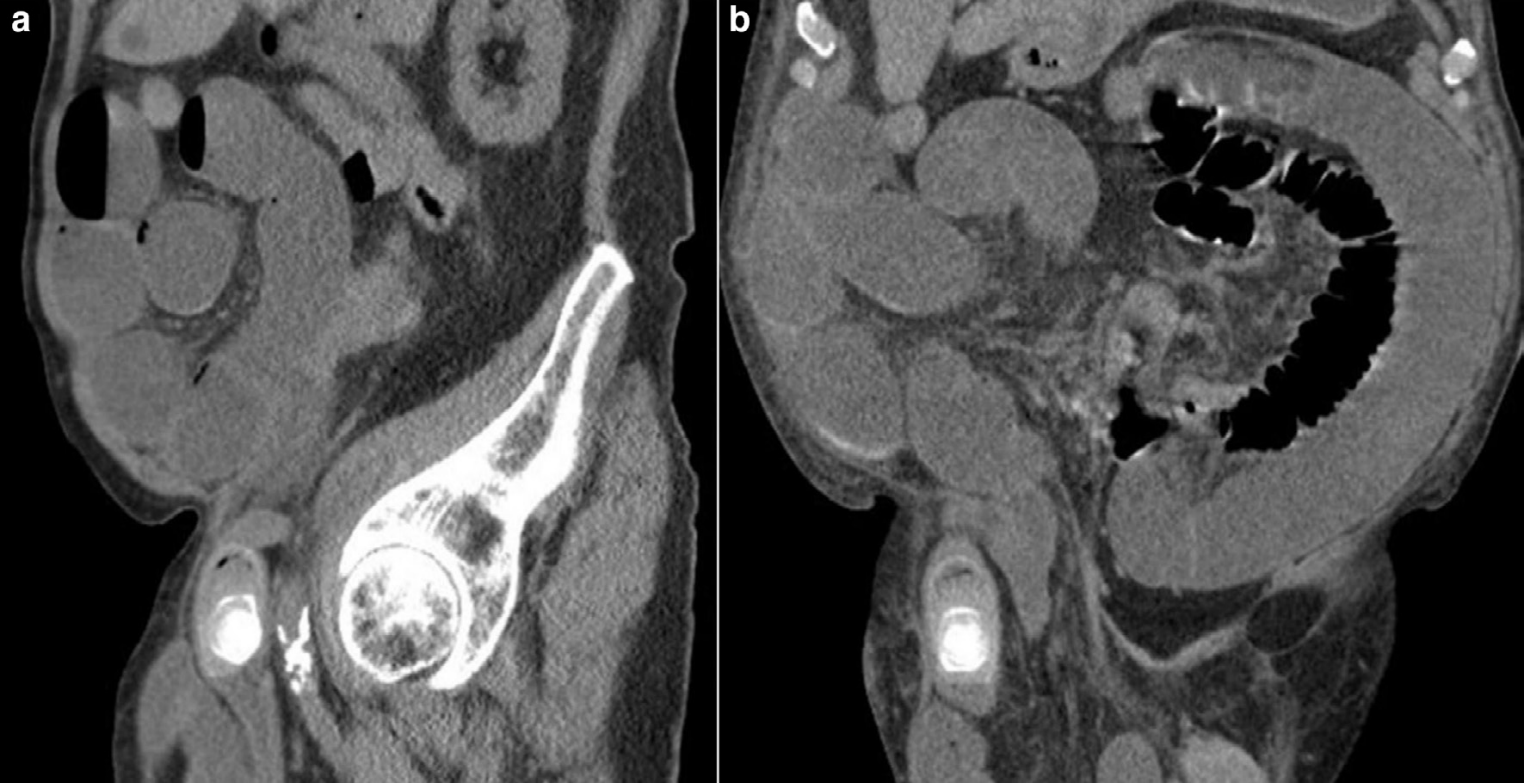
(a, b) Sagittal and coronal sections of the presentation unenhanced CT study showing a large gallstone lodged at the neck of the right inguinoscrotal hernia. Dilated loops of upstream small bowel are demonstrated within the abdominal cavity and within the hernial sac.
